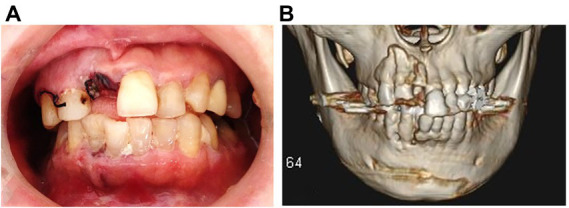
(A) Intraoral view at the patient’s first visit (5.7.2012). (B) Three-dimensional computed tomography scan obtained before treatment (5.7.2012).
